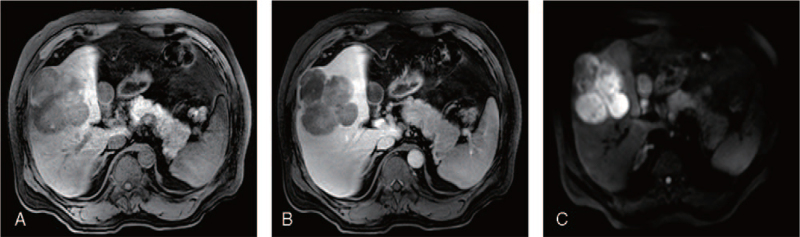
Magnetic resonance imaging (MRI) of the liver, gallbladder, and spleen. (A) T1 weighted (T1W1) image; (B) T2 weighted (T2W1) image; (C) diffusion weighted imaging (DWI).
